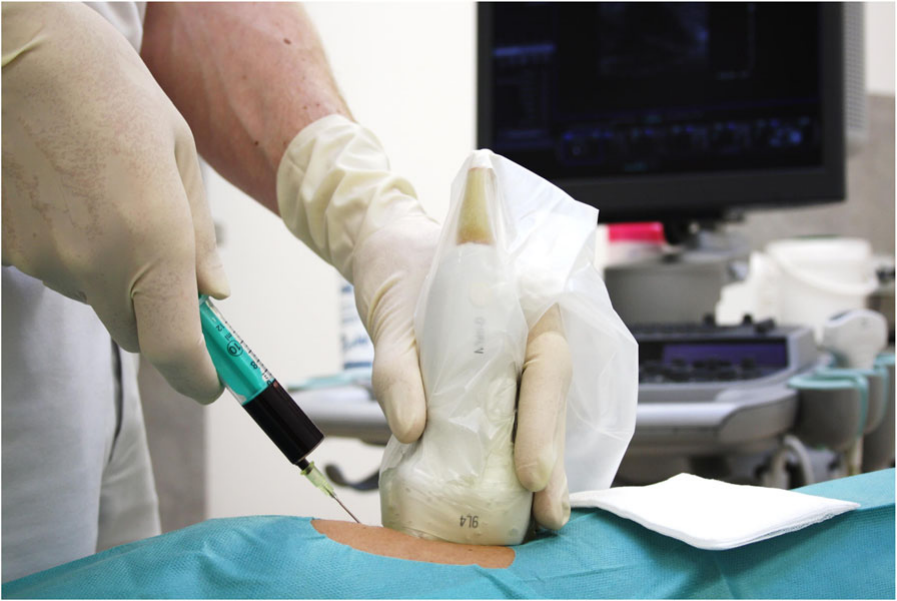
Ultrasound guided puncture of a haematic lesion in context of a structural muscle injury of the rectus femoris muscle
